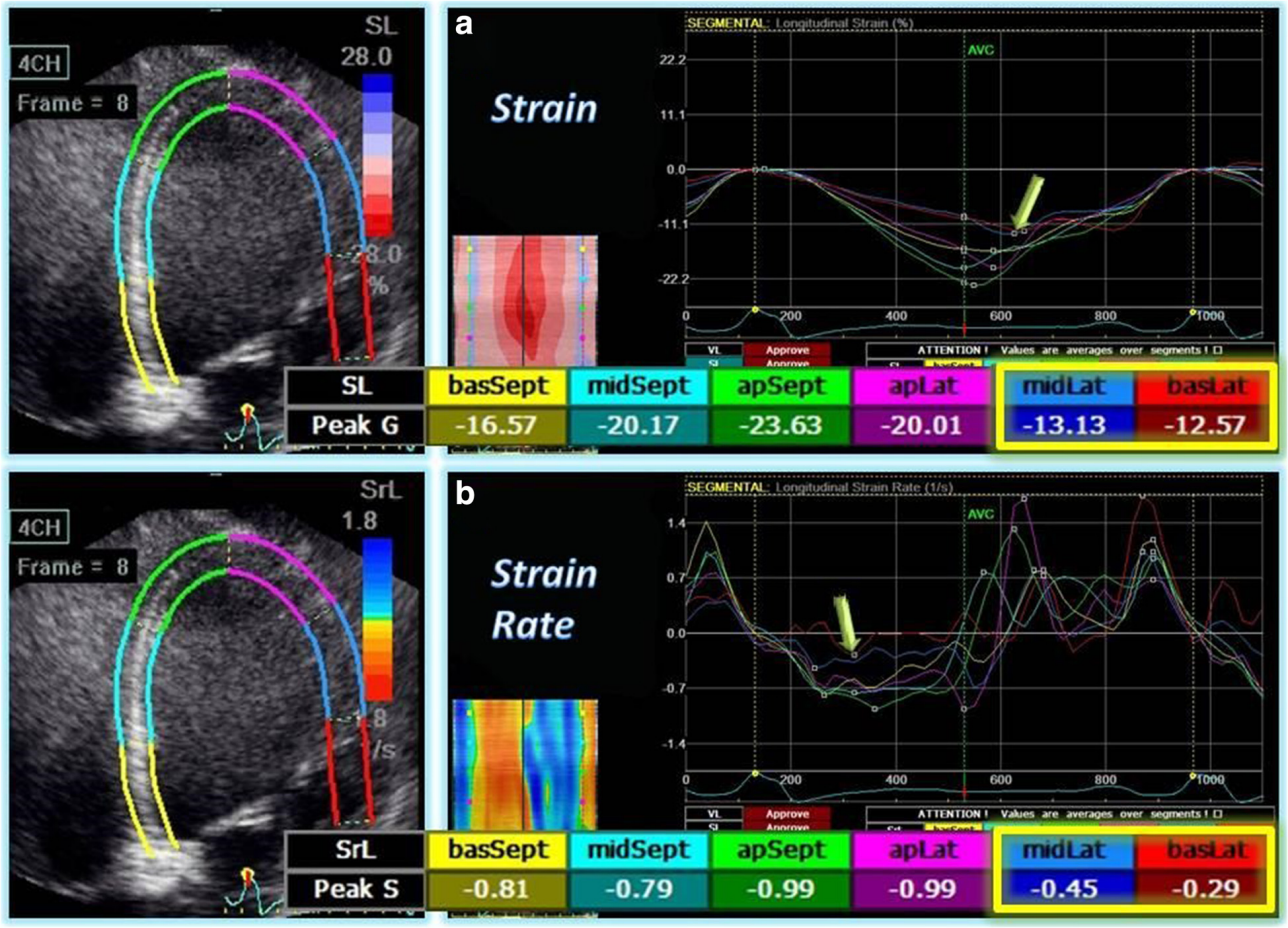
Example of a STE analysis. Patient with CD and normal LVEF. Analyzes of strain (a) and strain rate (b) in a patient with CD and preserved LVEF, apical four-chamber view. Ventricular myocardium is divided in six color-coded segments and strain/strain rate curves are displayed. Also, small boxes display color m-mode. Note a lack of deformation (worse values; yellow arrows) at the base and mid-segment of lateral wall (red and blue curves, respectively), classically affected by CD
